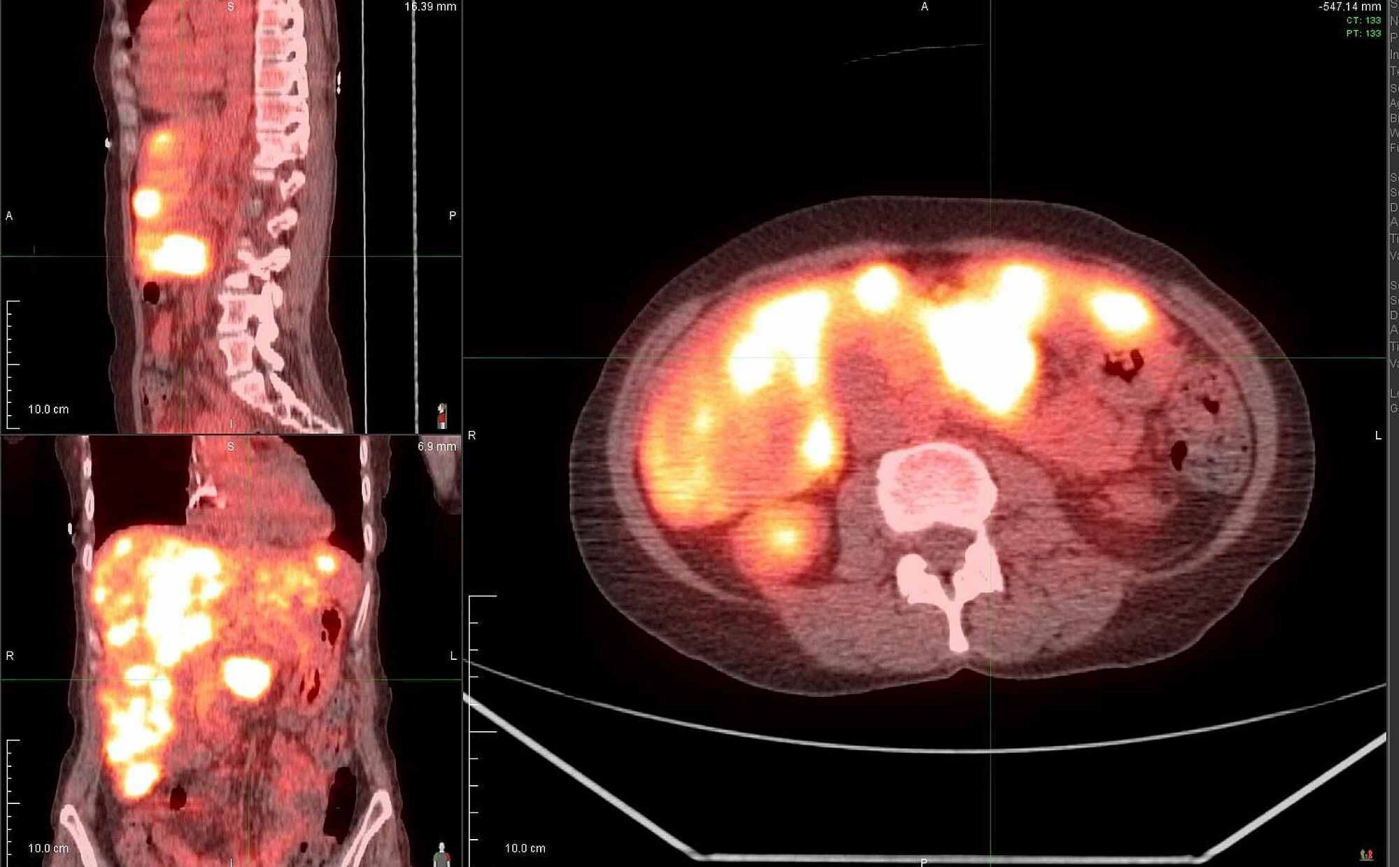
PET-CT demonstrating pancreatic adenocarcinoma with metastases to the liver, prior to the initiation of treatment
